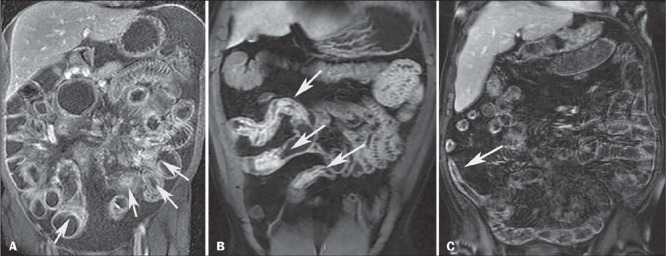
A: Contrast-enhanced coronal T1-weighted MRE sequence with fat suppression, showing
